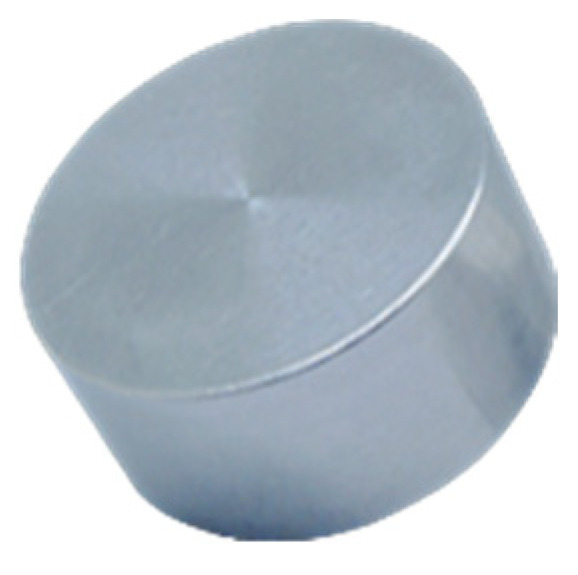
Picture of ultrasonic transducer.
